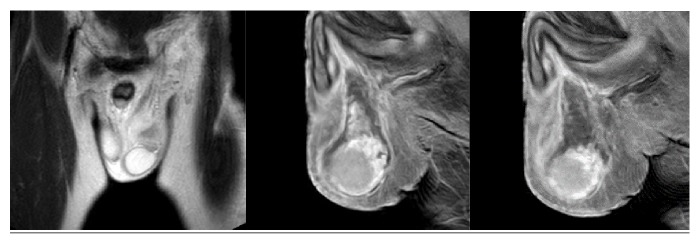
MRI of the scrotum which confirms the presence of hematoma. There is no contrast enhancement of the collection.
